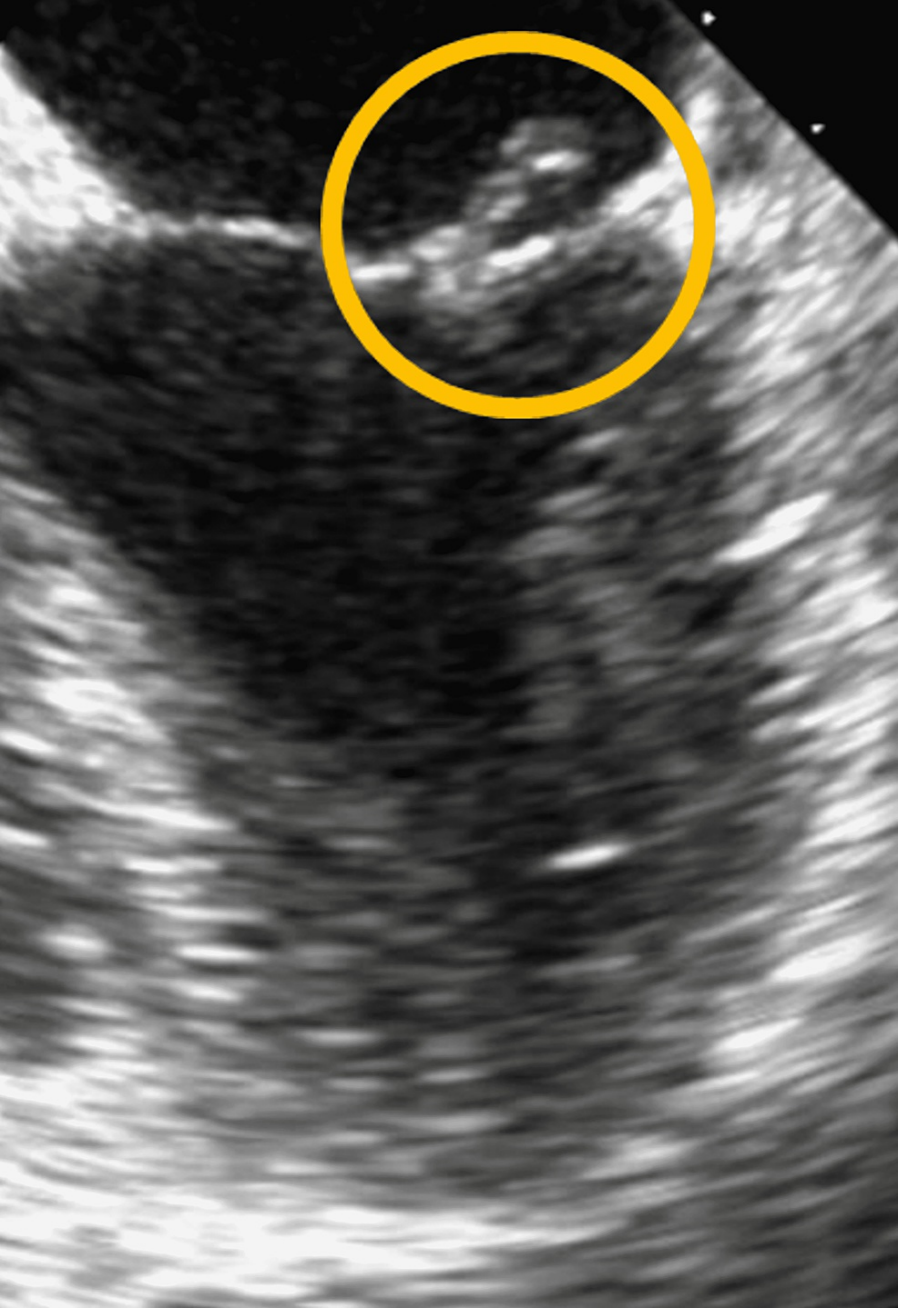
A transesophageal echocardiogram performed early during the patient’s hospitalization showed a hypermobile vegetation along the anterior mitral valve leaflet (circled) confirming suspected infective endocarditis.
